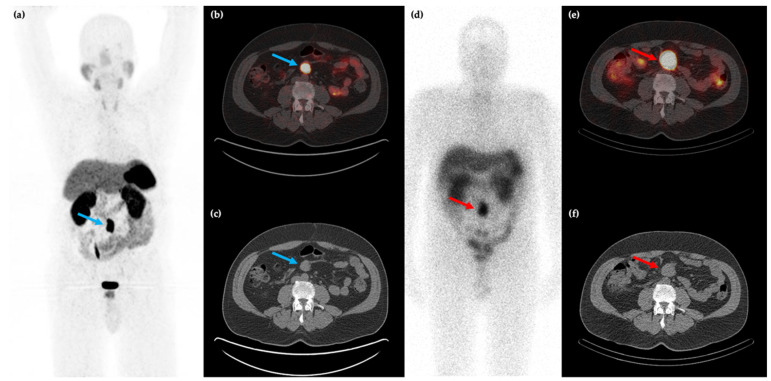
58-year-old male with a history of rectal bleeding and a mesenteric mass identified on conventional CT imaging. ^68^Ga-DOTATATE PET/CT maximum intensity projection (MIP) (a) and axial (b,c) images shows the somatostatin receptor (SSTR) positive lesion at the root of the small bowel mesentery (blue arrows) with improved spatial resolution compared to ^111^In-pentreotide SPECT/CT ((d–f), red arrows).
